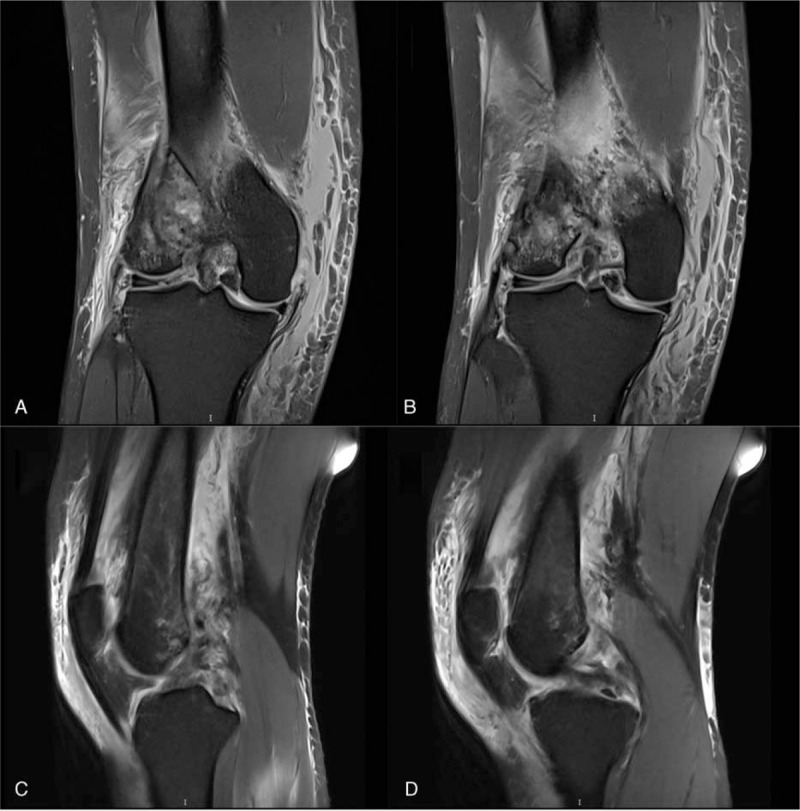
(A–D) The preoperative MRI images of the knee clearly showed medial and lateral collateral ligaments, anterior and posterior cruciate ligaments suffered varying degrees of injury.
